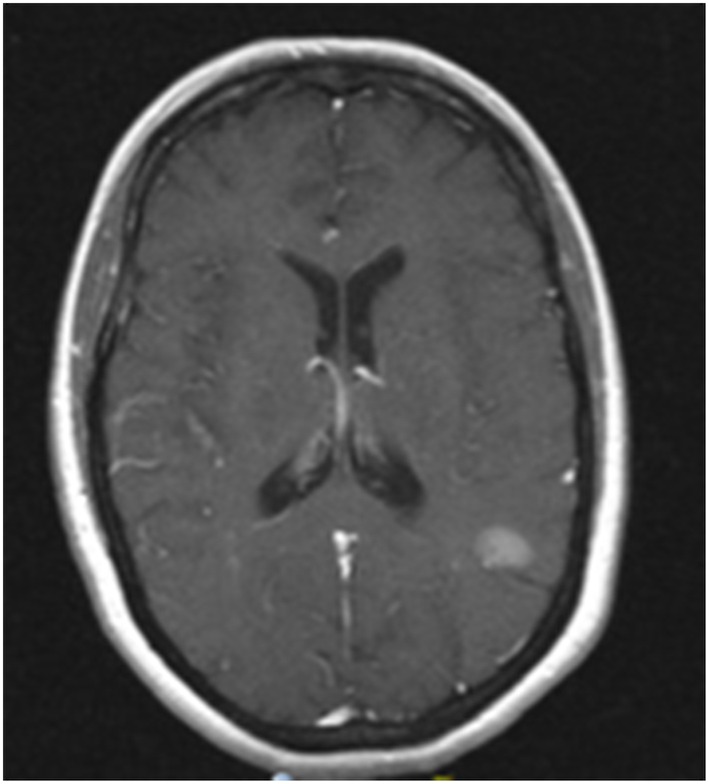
MRI image of progression of left temporoparietal metastasis. Contrast enhanced T1 MRI showing a 1.6 cm left temporoparietal lesion that increased in size after completion of whole brain radiation. This lesion was treated subsequently with stereotactic radiosurgery.
